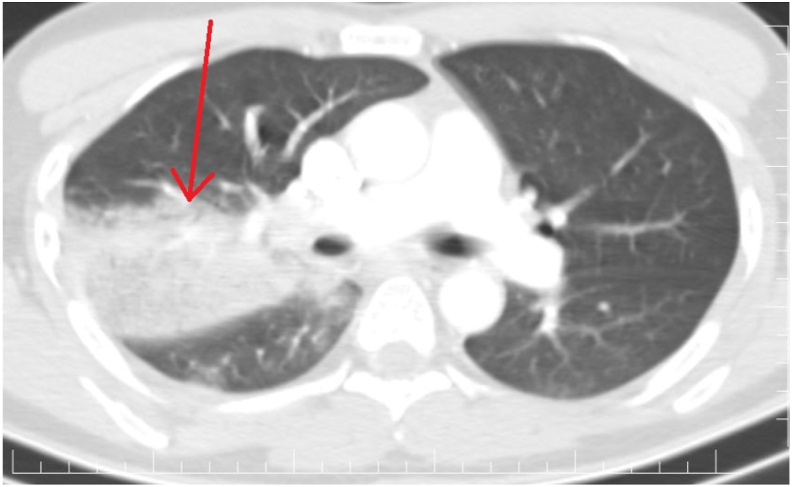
Chest CT scan with contrast parenchymal window showing consolidation in the upper lobe of the right lung (arrow).
